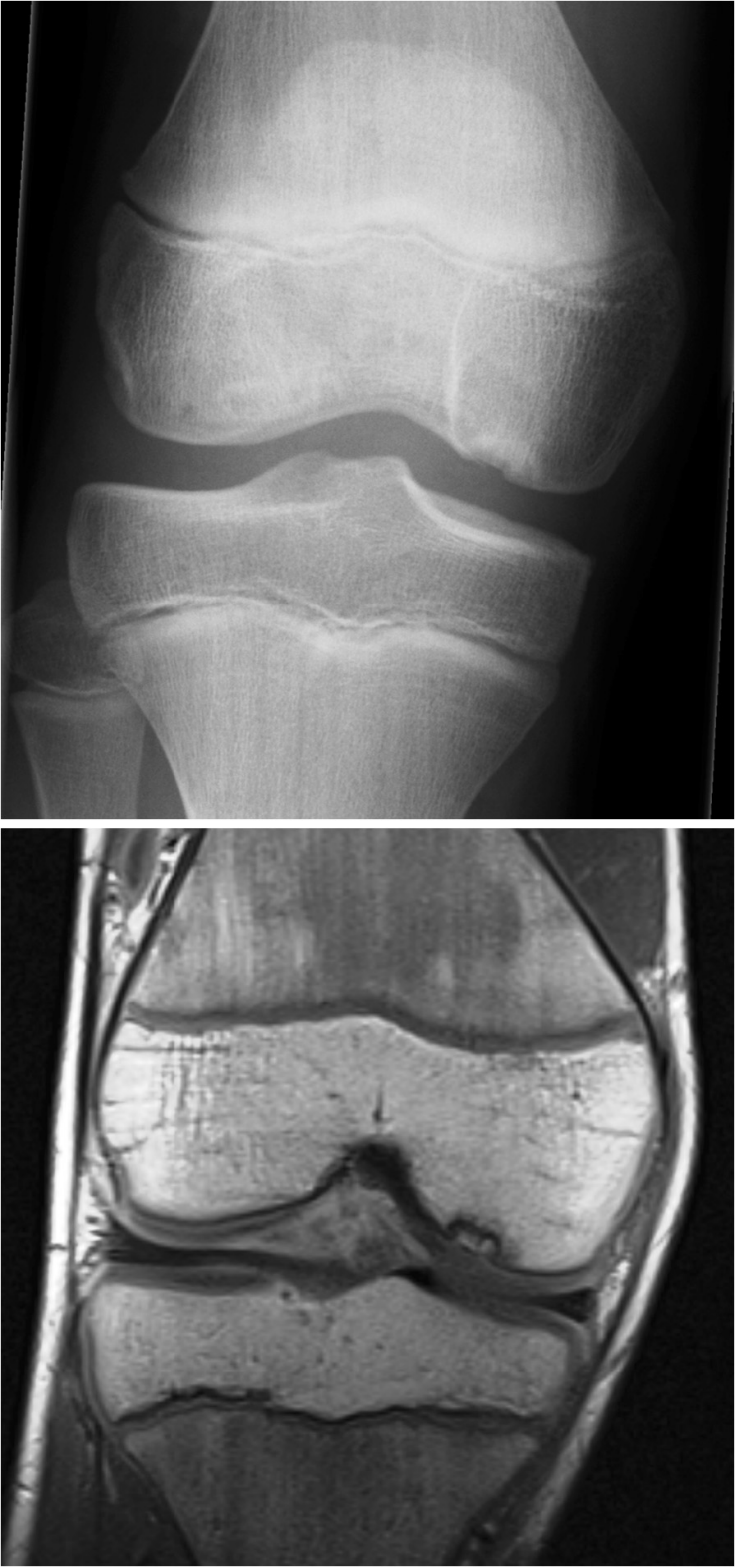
Radiograph and MRI showing an osteochondral lesion of medial femoral condyle.
